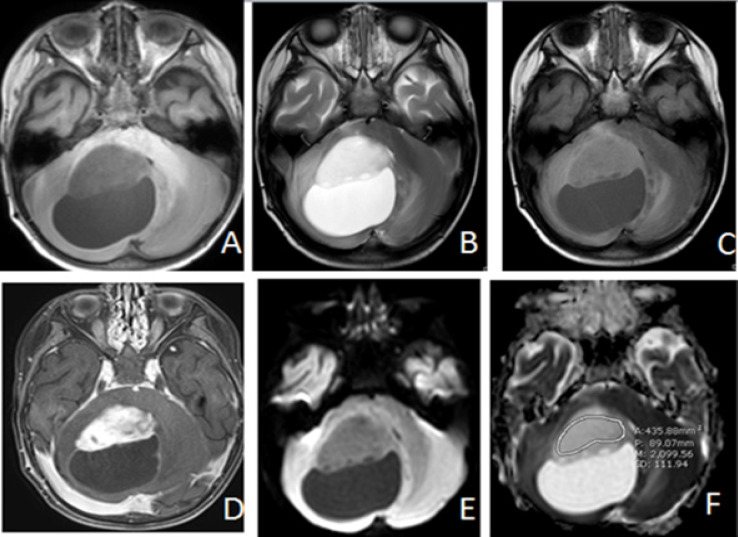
Pilocytic Astrocytoma (WHO Grade I) in 2 Year-Old-Boy. Axial T1W(A), T2W(B), FLAIR(C), Post contrast T1WFS(D), DWI (E) and ADC map (F) images representing case that showed hypointense solid part of the tumor with visual scale 2 on DWI(E) and ADC value measurement = 2099.56 X 10^-6^ mm^2^/s on ADC map (F) which is much higher than the cutoff value
